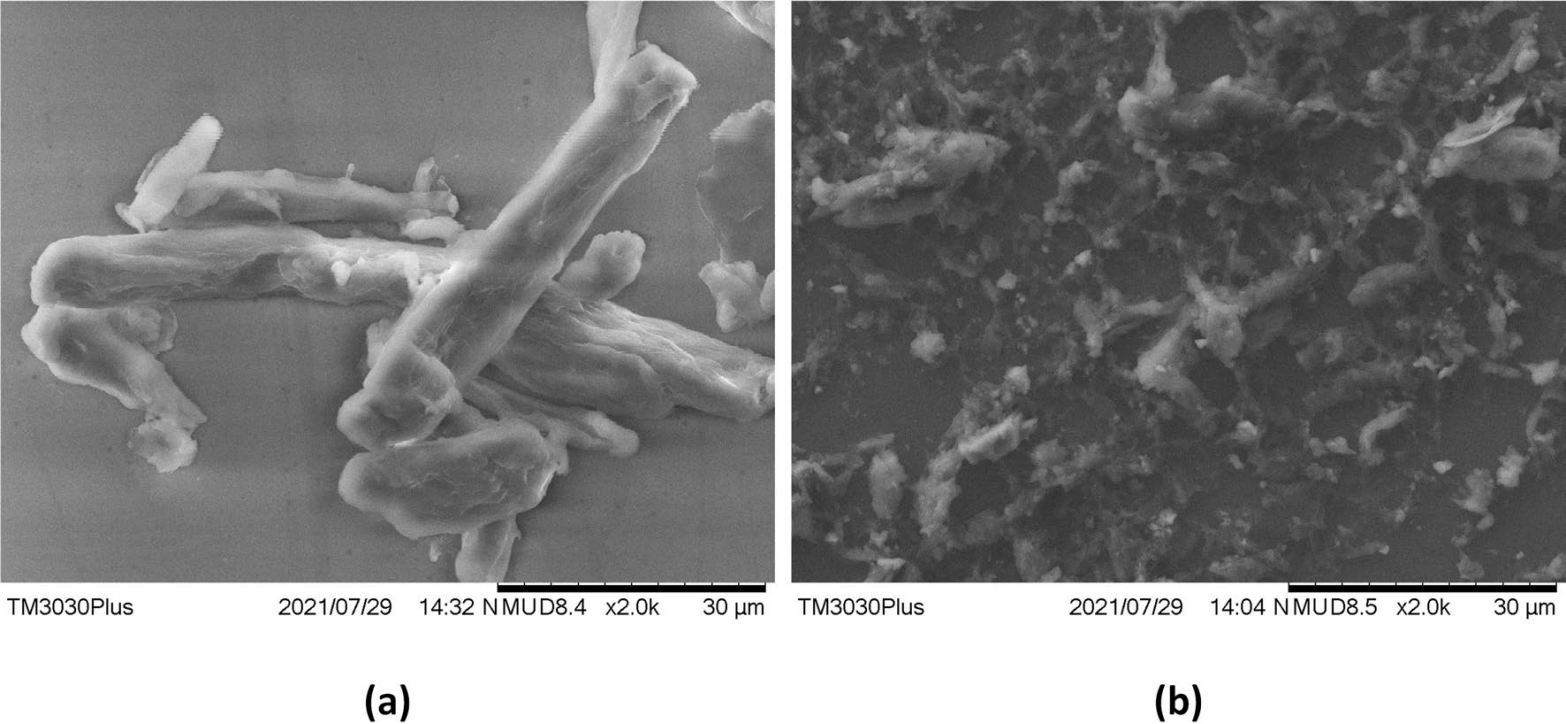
SEM images of cellulose decorated sensing layer. (a) Decorated cellulose before ultrasonication. (b) Decorated cellulose after ultrasonication.
